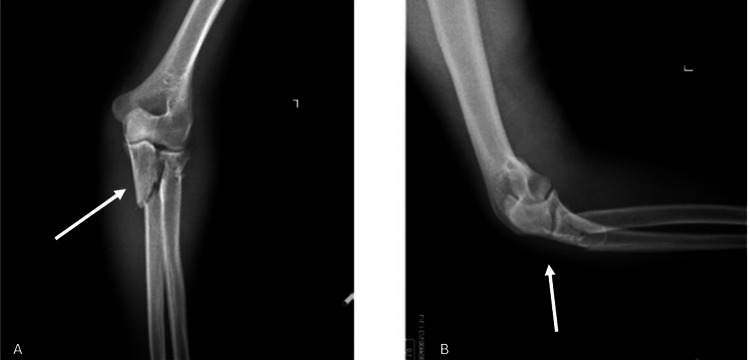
X-ray images of the patient at hospital admission. Both anteroposterior (A) and lateral (B) X-ray images confirm a fracture at proximal ulna and radius White arrows indicate the fracture site at proximal ulna and radius.
